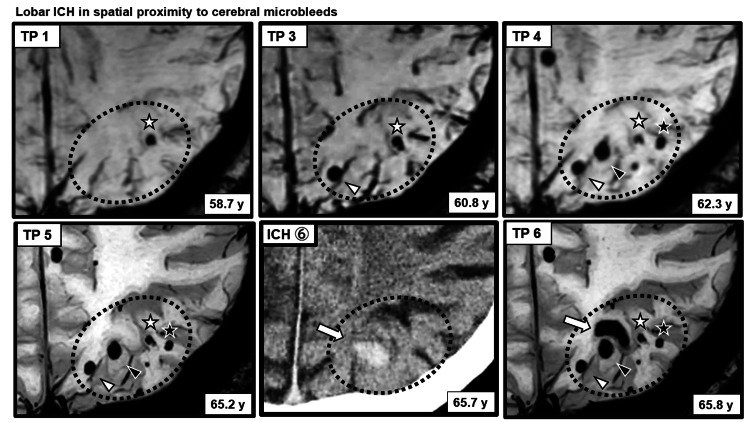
ICH arising in close spatial proximity to CMBs in a lobar region New formation and clustering of CMBs in the left parieto-occipital region (outlined by a dotted ellipse) and a subsequent ICH arising in close proximity are shown. Among the two CMBs located closest to the hemorrhagic focus, dynamic morphological changes were observed prior to ICH onset: one CMB showed enlargement (black arrowhead), whereas the other exhibited irregular deformation with a reduction in size (white asterisk). In contrast, two more peripheral CMBs (white arrowhead and black asterisk) did not demonstrate apparent size changes. The location of the corresponding ICH was confirmed on CT images shown in Figure 1. CMBs: cerebral microbleeds; ICH: intracerebral hemorrhage; SWI: susceptibility-weighted imaging; CT: computed tomography
